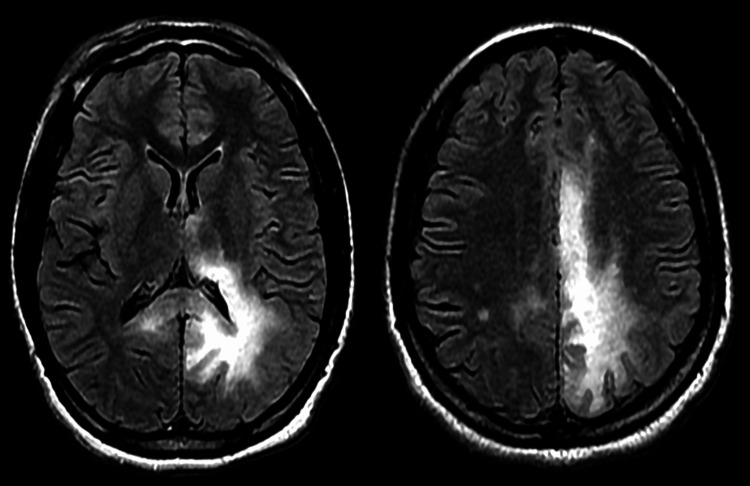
MRI of diffuse T2 FLAIR showed hyperintensity involving the left corona radiata, parietal, occipital region, and thalamus/basal ganglia FLAIR: fluid-attenuated inversion recovery
